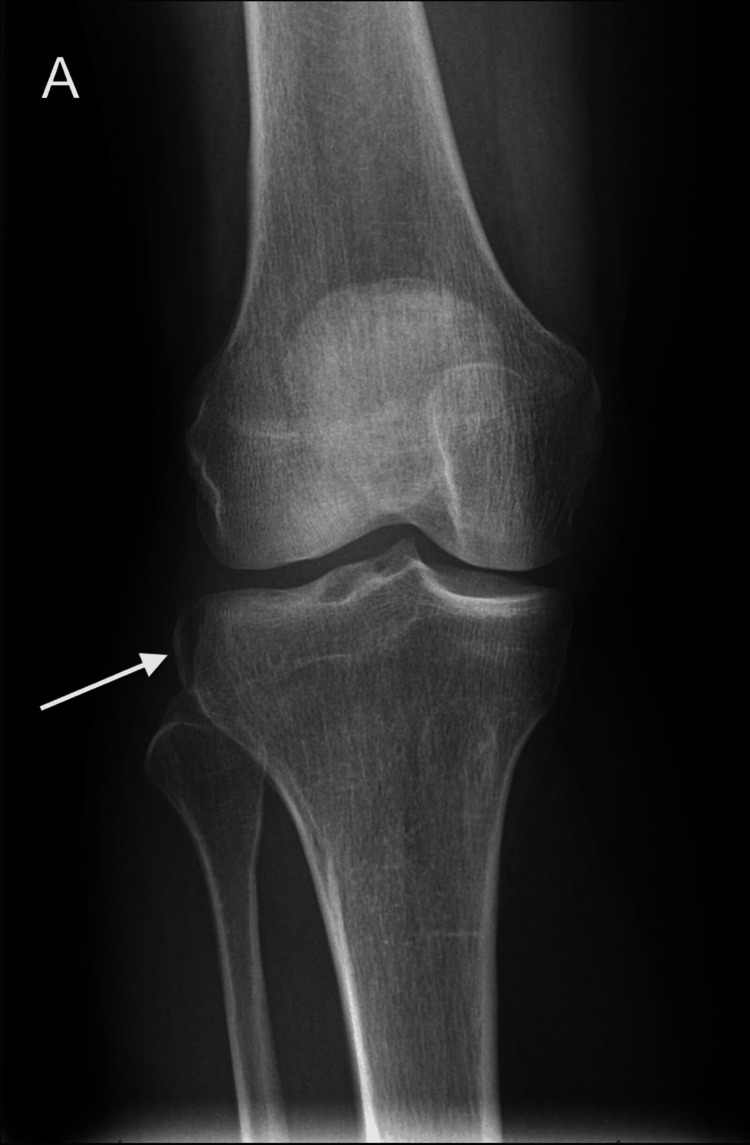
(A) Anterior-posterior X-ray view of the right knee shows an avulsion fracture of the lateral tibial plateau (Segond fracture) with minimal displacement (arrow).
